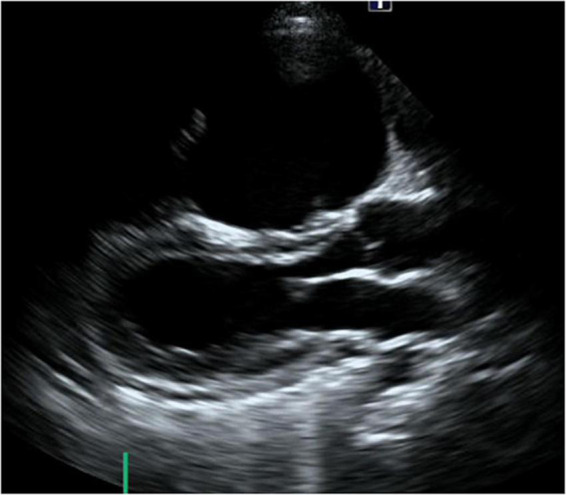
Long-axis view at end-diastolic phase of transthoracic echocardiography in Case 3. Note that the right ventricle is much larger than the left ventricle, and the left ventricle is very small (end-diastolic dimension 31 mm).
